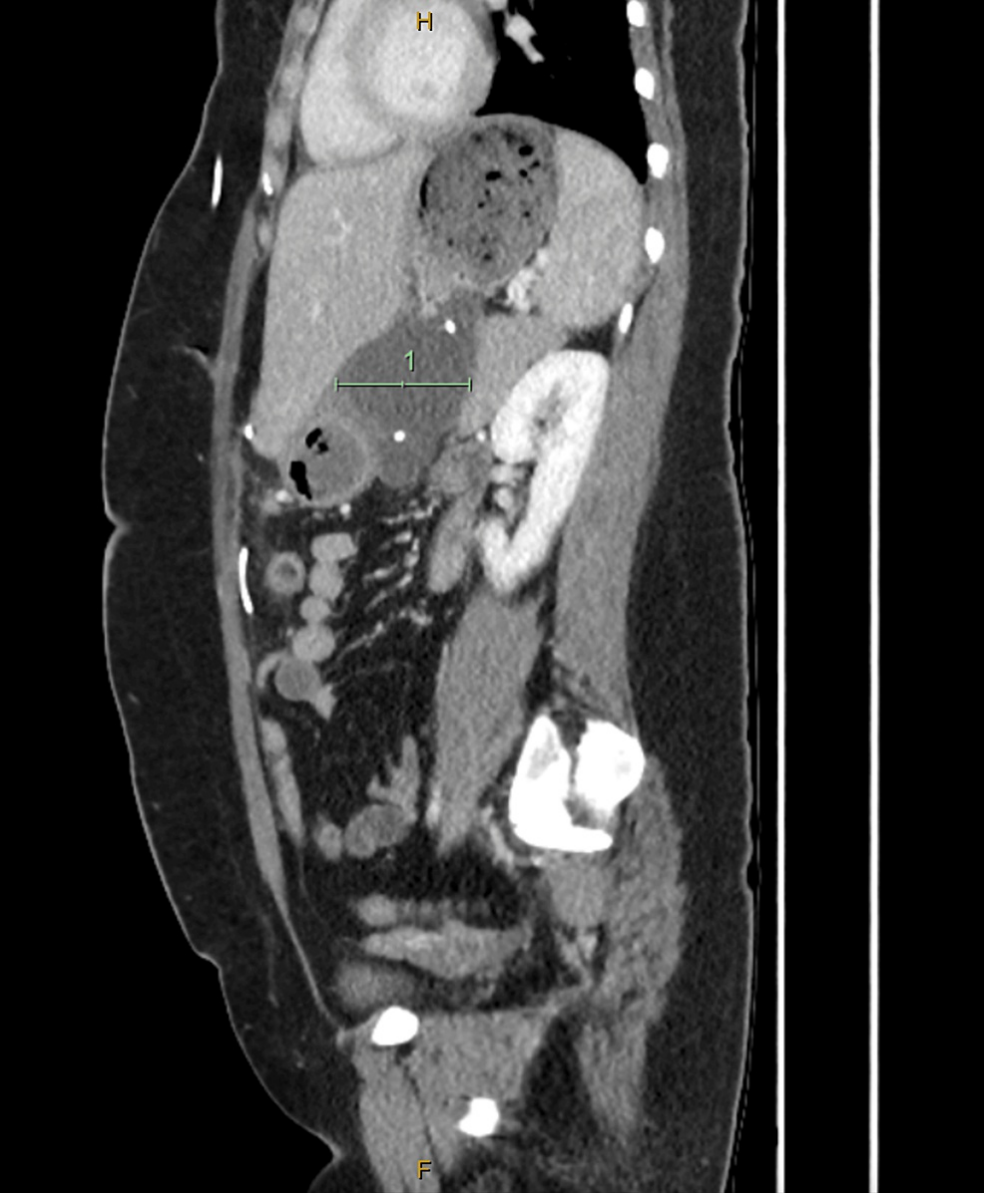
CT sagittal view showing collection within the lesser sac
